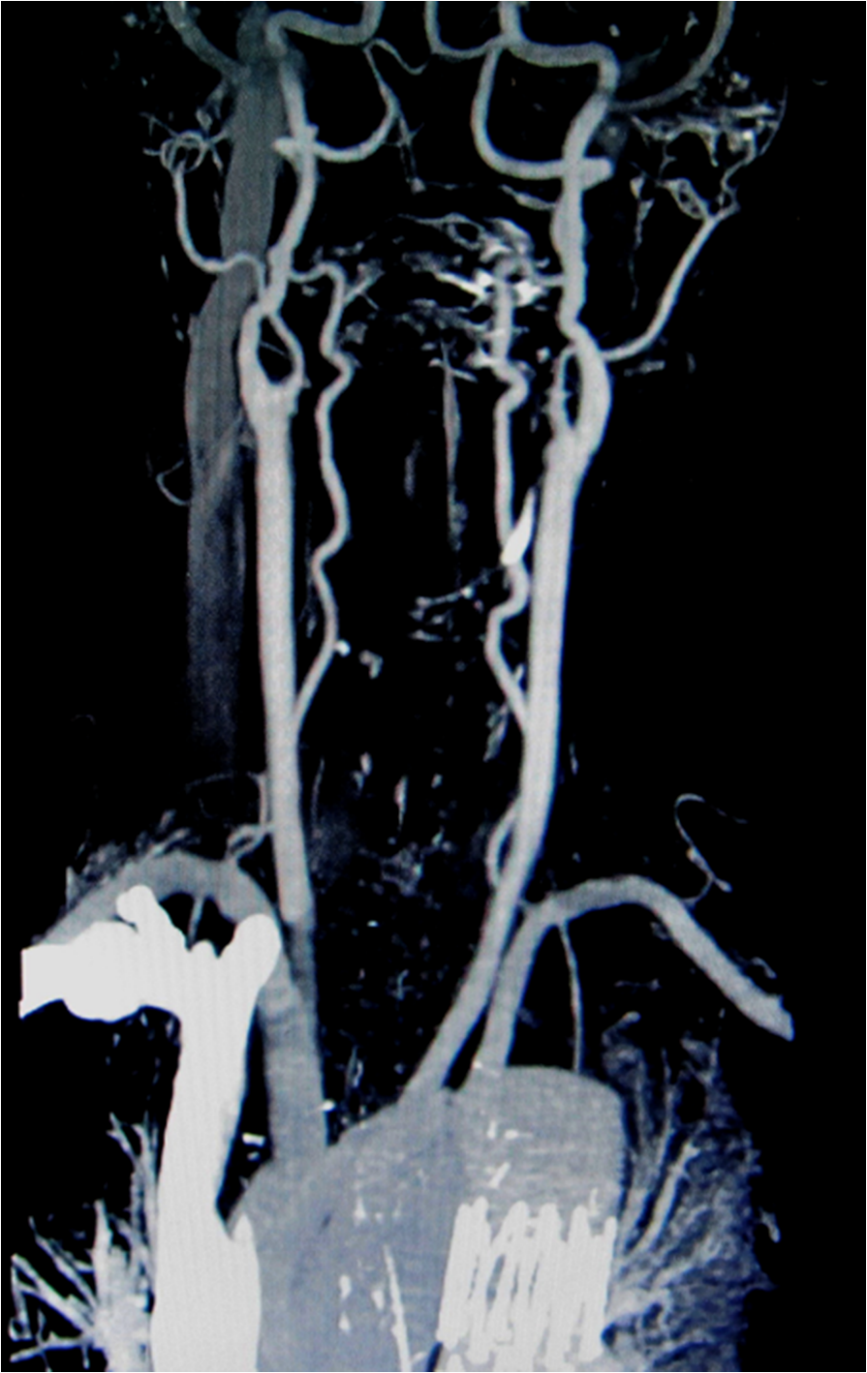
Postoperative 3-D reconstructed computed tomography scan. No abnormal vessels or aneurysms were detected in the neck.
